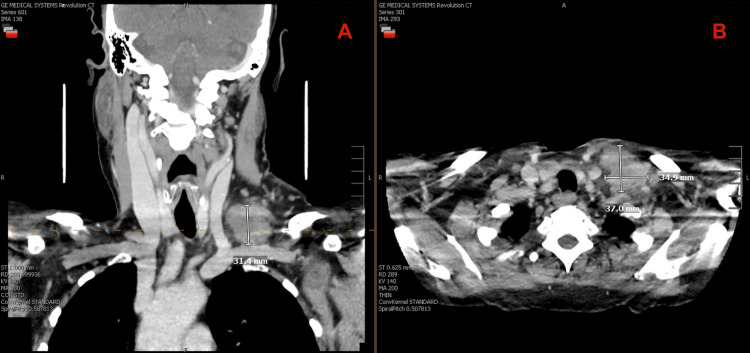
Contrast-enhanced CT of the neck obtained September 12, 2024, prior to biopsy. Coronal (A) and axial (B) views show a left supraclavicular mass measuring approximately 37 × 35 mm, displacing adjacent vascular structures without compression or hemorrhage.
